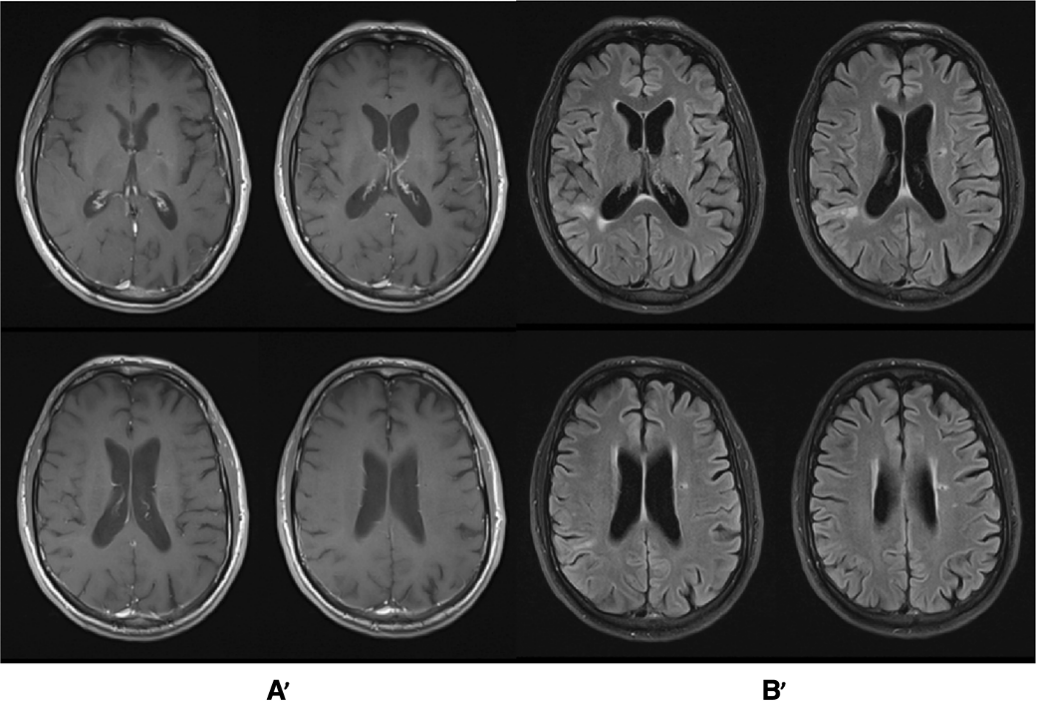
Follow-up brain magnetic resonance imaging
